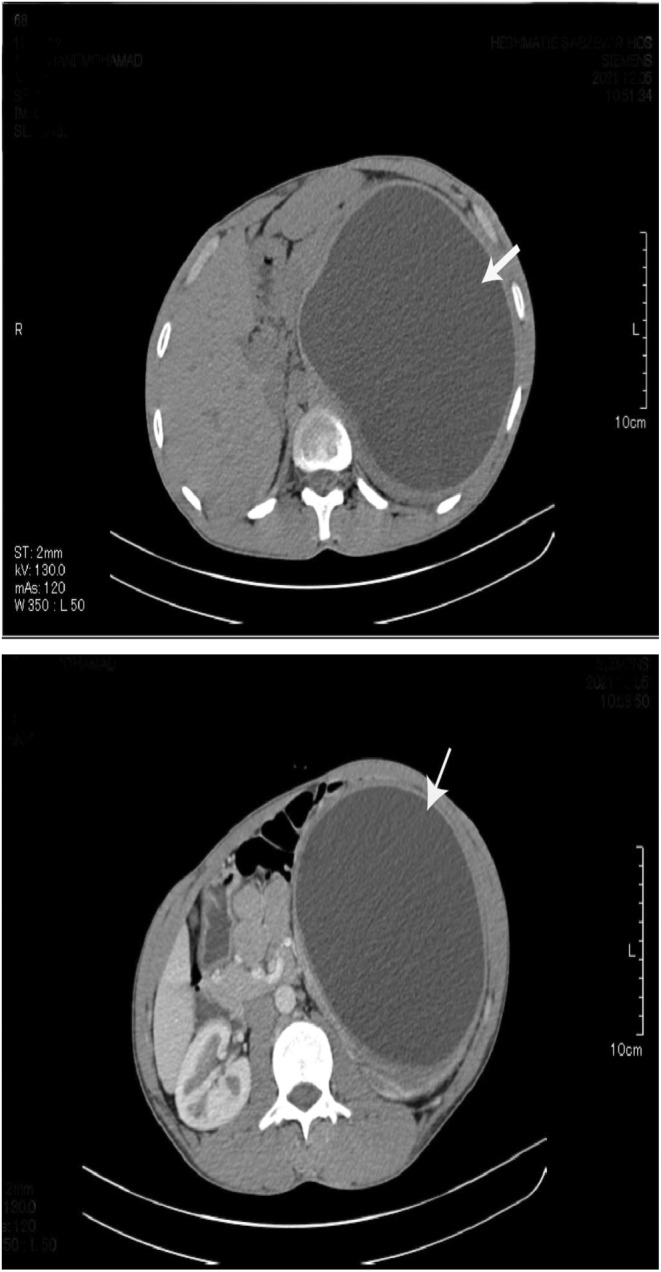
Axial sections of abdomen CT scan without IV contrast
